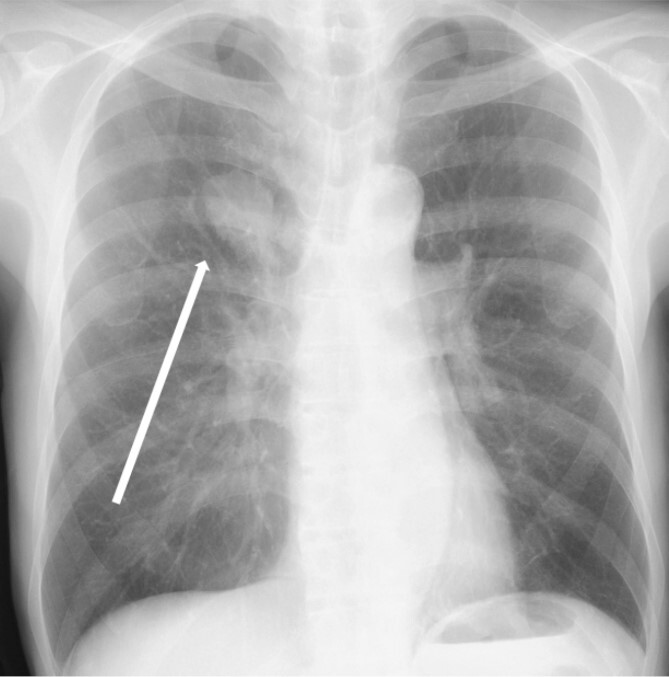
Chest X-ray findings (frontal view). Mass shadow in the upper lobe of the right lung.
